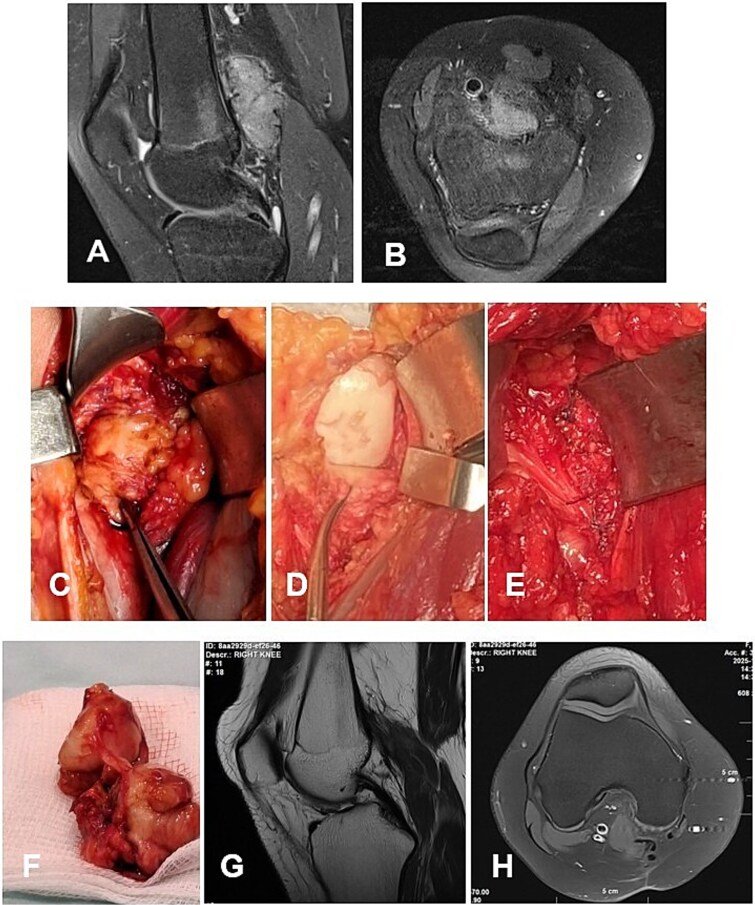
Case 4. (A and B) Preoperative Sag and Ax MRI TSE PD FS—a lobulated intra-articular neoplasm with a posterior mediocentral localization, displacing the neurovascular bundle laterally. (C and F) Macroscopic appearance of the N-TGCT measuring 3 × 1 × 2 cm, *in situ* and after excision. (C) Posterior synovectomy performed with lateral retraction of the *m. gastrocnemius medialis.* (D) The joint after synovectomy, showing grade I chondral changes according to the Outerbridge classification. (E) Reconstructed fibrous capsule. (E and F) Postoperative 12-month sagittal and axial MRI (TSE PD FS) demonstrates no evidence of recurrence of the resected neoplasm. (G and H) PD FS, proton density weighting with fat suppression; TSE, turbo spin echo.
